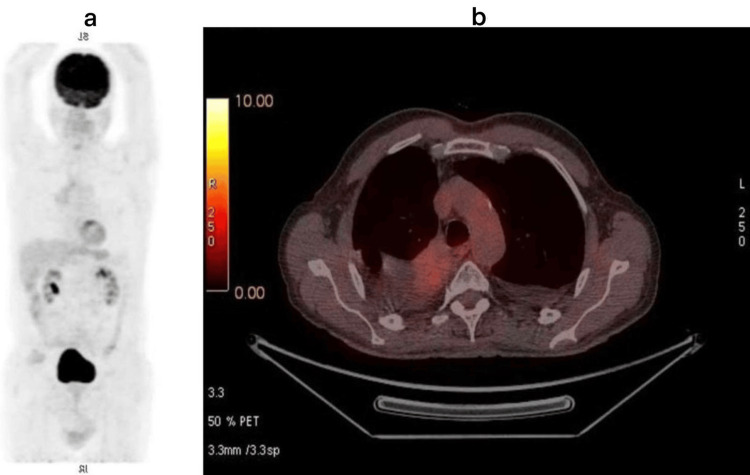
Images from the last FDG-PET/CT showing a complete response (a: coronal view; b: axial view) FDG: fluorine-18 fluorodeoxyglucose
